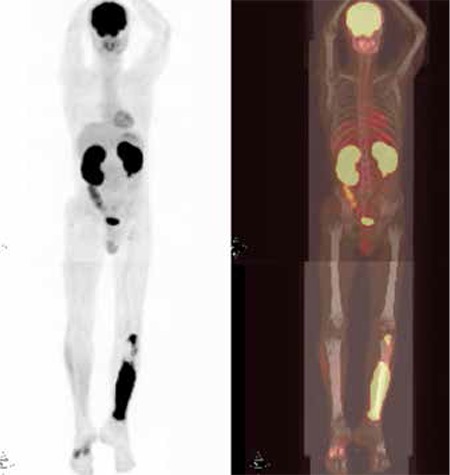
Thirty six-year-old male patient with pathologic fracture of the lefttibia underwent intramedullary curettage. Biopsy confirmed DLBCL. Patientunderwent FDG PET/CT scanning for staging. Maximum intensity projection(MIP) (left) and FDG PET/CT fusion (right) images demonstrated very intenseFGD accumulation (SUVmax:17.1) extending to soft tissue at the 2/3 distalpart of the left tibia and intense tracer uptake at the proximal metaphysisof the left tibia which were both compatible with disease involvement.Additionally, there was very intense diffuse FDG uptake in bilateral enlargedkidneys which was suspicious for primary disease involvement.
